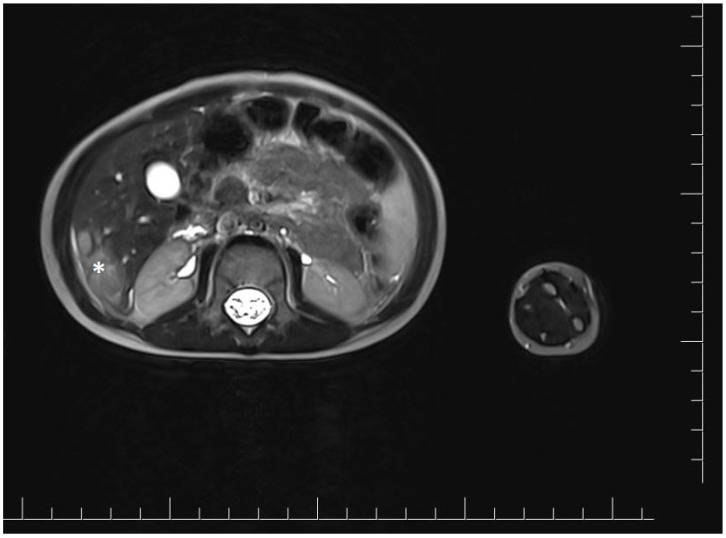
Magnetic resonance imaging of the abdominal cavity showing lesions in the right hepatic lobe after two cycles of cisplatin chemotherapy. The tumor is marked with an asterisk.
